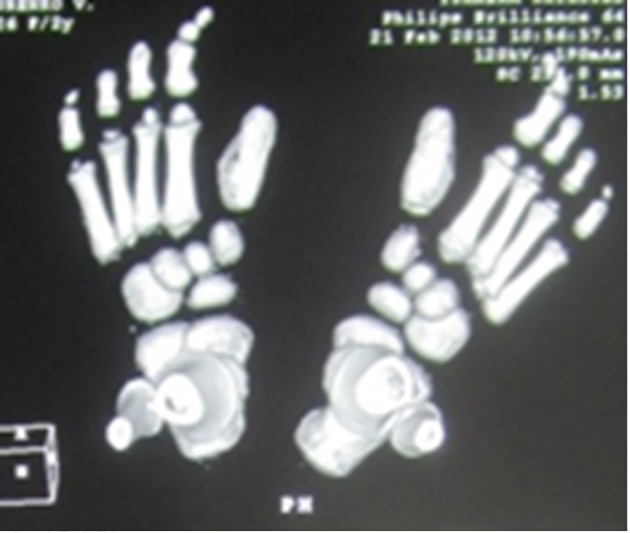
Reformatted CT scan in a 14-year-old girl showed monophalangia with subsequent development of severe shortening of the first metatarsals (secondary to abnormal fusion of the epiphyses).
